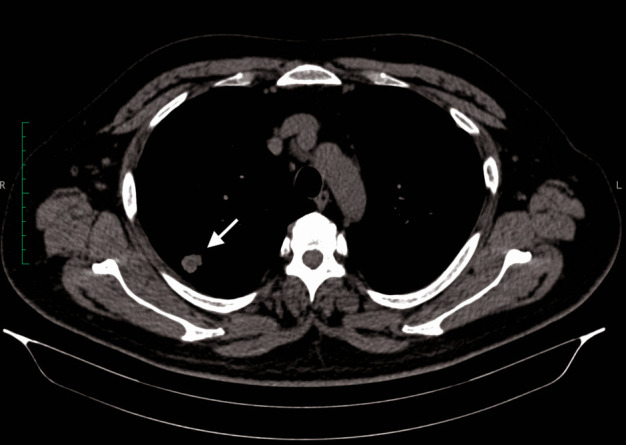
Lung CT-scan: solitary pulmonary nodule situated in the posterior segment of the right lung’s superior lobe (white arrow)
